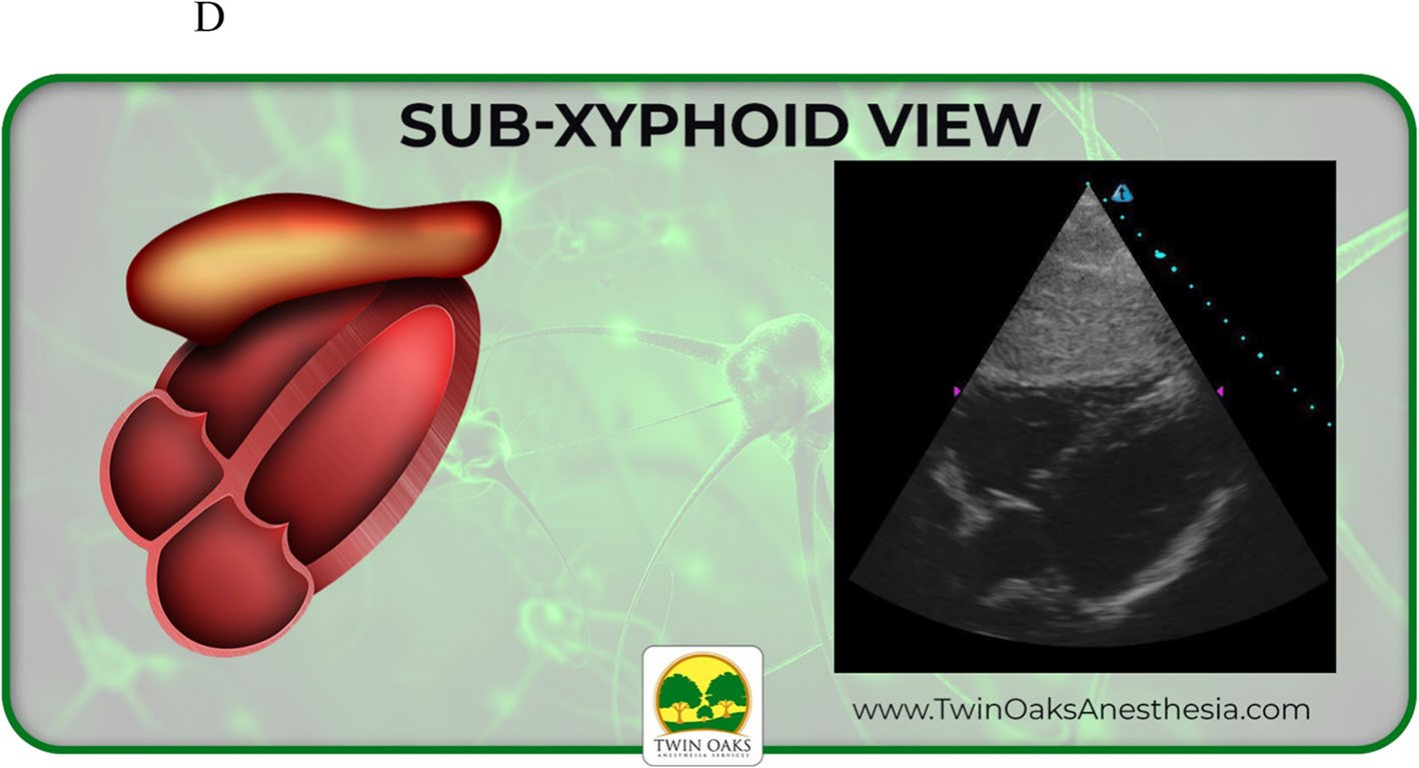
Study graphic and corresponding sonogram of sub-xyphoid TTE view
